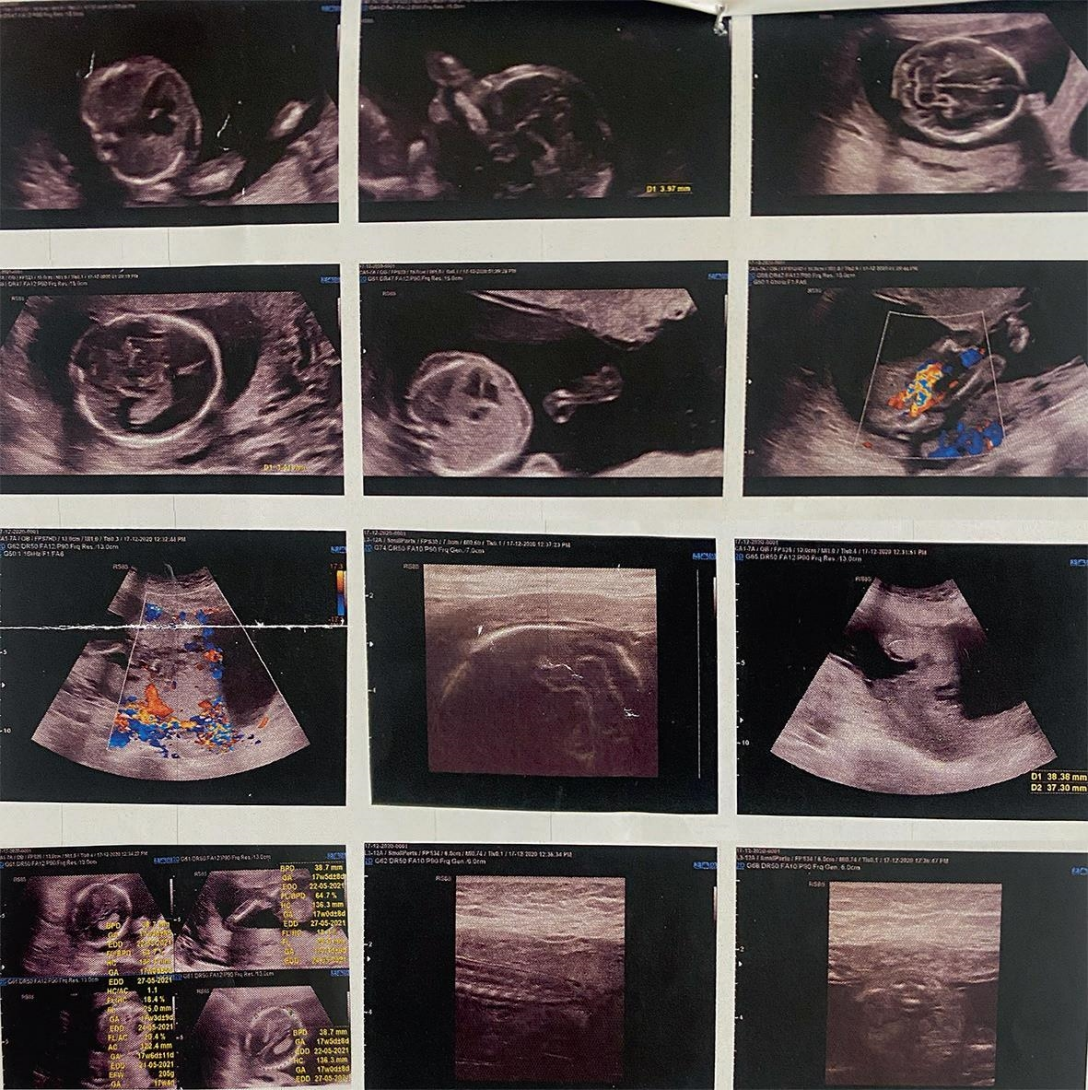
Ultrasound images obtained at 17 weeks and 5 days of gestation demonstrating anterior placenta previa with features of placenta accreta spectrum and suspected placental invasion of the bladder wall on color Doppler imaging.
